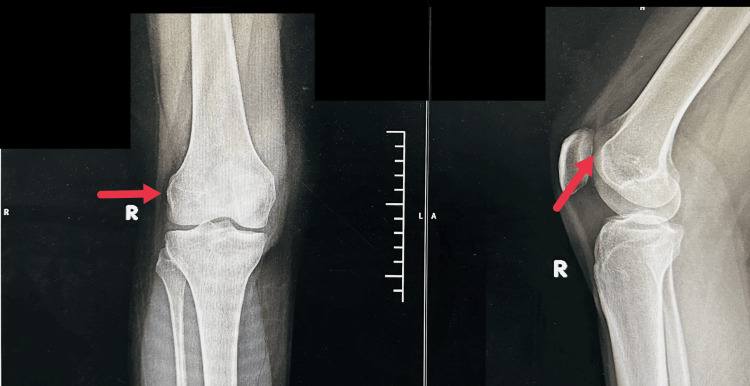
Preoperative X-ray of the right knee The red arrow shows a doubtful lytic lesion on the lateral distal femoral condyle.
